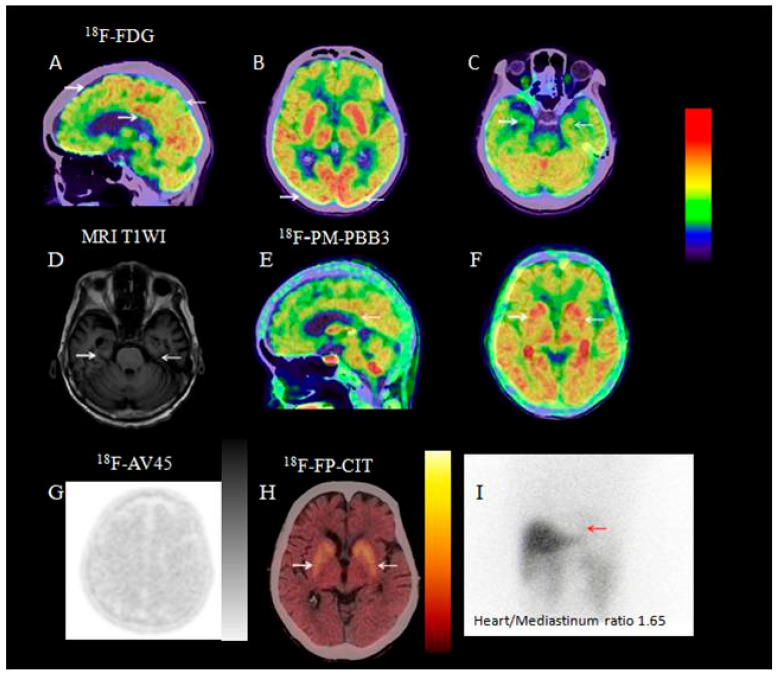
A 78-year-old female patient with 1-year bradykinesia, 6-month resting tremor, and 2-month neuropsychiatric symptoms. ^18^F-FDG PET/CT shows bilateral occipital and medial temporal hypometabolism, with preserved posterior cingulate metabolism ((A–C); white arrows). MRI T1WI shows bilateral medial temporal atrophy ((D); white arrow). ^18^F-PM-PBB3 PET shows tau deposition in the cerebral cortex and basal ganglia ((E,F); white arrows). ^18^F-AV45 PET shows cerebral Aβ deposition (G). ^18^F-FP-CIT PET/CT shows reduced bilateral basal ganglia dopamine transporter distribution, more severe on the right ((H); white arrow). ^131^I-MIBG scintigraphy shows a heart/mediastinum ratio of 1.65 ((I); red arrow), indicating cardiac sympathetic denervation. (Note: The images related to this case are adapted from Figure 1 in the author’s previously published literature [14]. In this study, the presentation order of the images has been adjusted as the patient’s case was included for analysis, while the core content of the images remains consistent with the original and has not been modified.).
